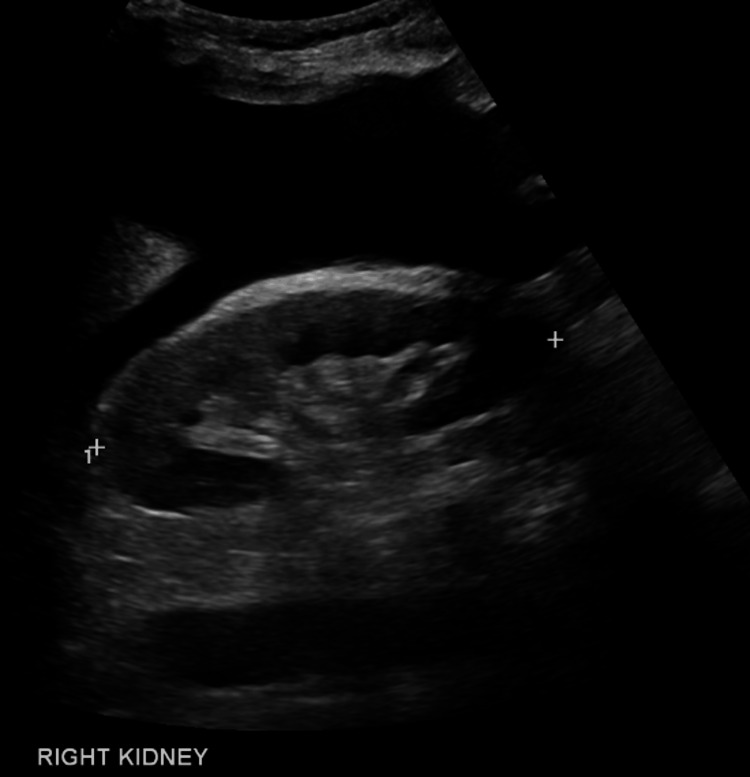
Right Renal Tract Ultrasound The right kidney showing no hydronephrosis with a moderate volume of ascites
